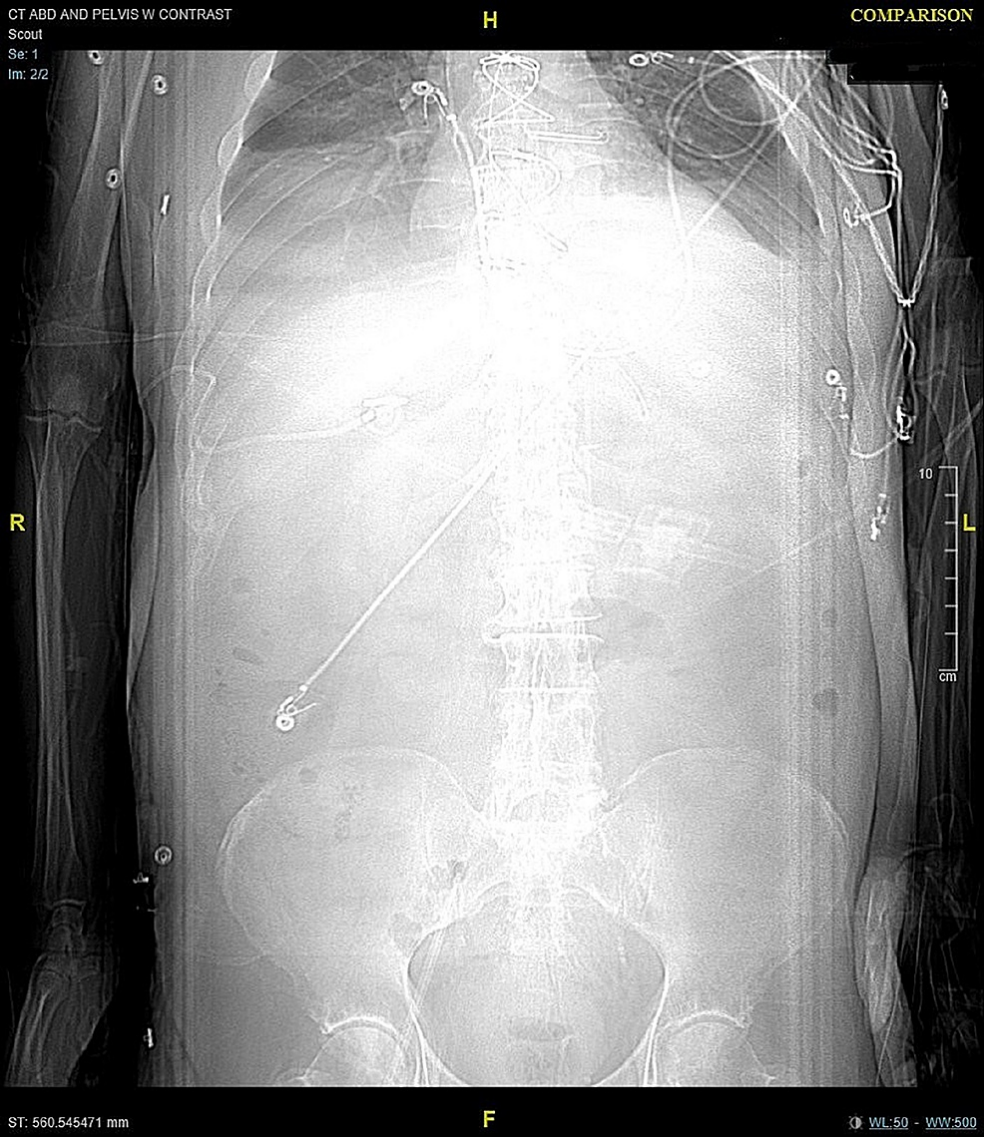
CT of the abdomen/pelvis on admission-scout. Scout: On admission, without abnormalities. CT: computed tomography
